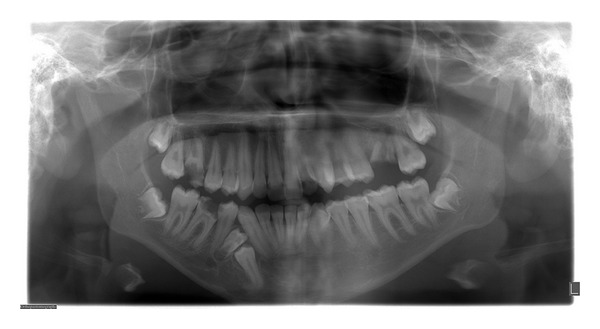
Orthopantomograph showing unilateral impacted partially formed and fused double premolars associated with, but separate from an impacted premolar.
